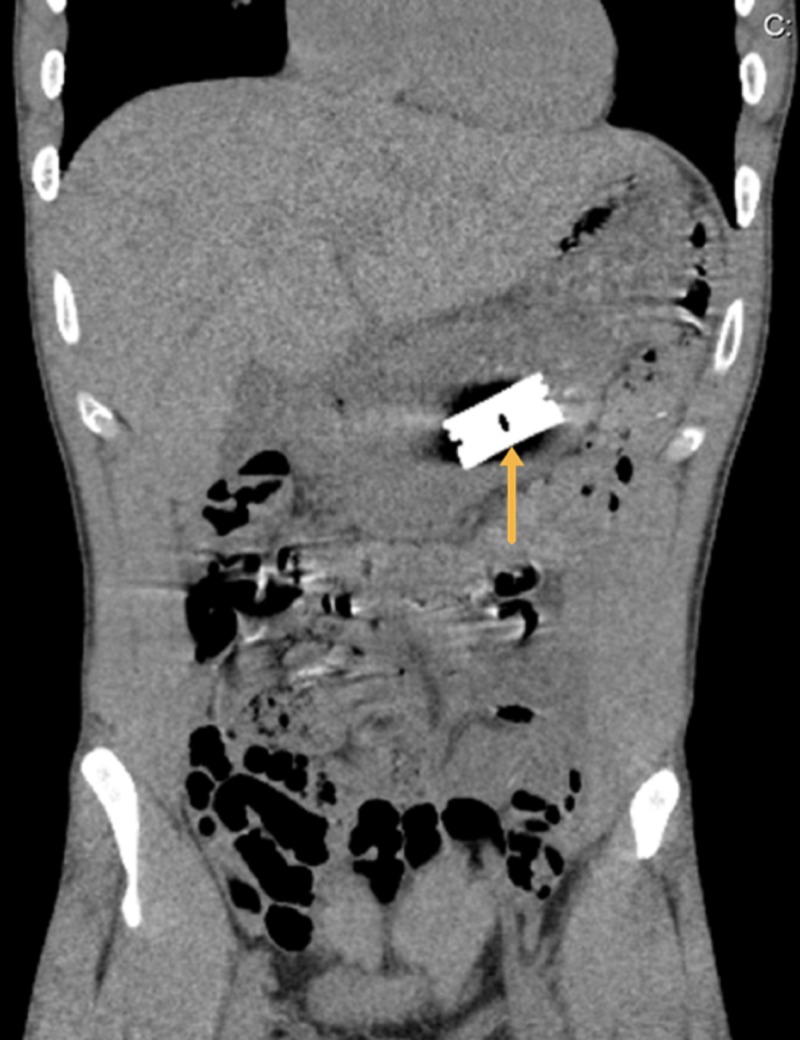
Computed tomography scan of the abdomen identifying the razor blade within the lumen of the stomach. Arrow demarcates the precise location of the razor blade.
